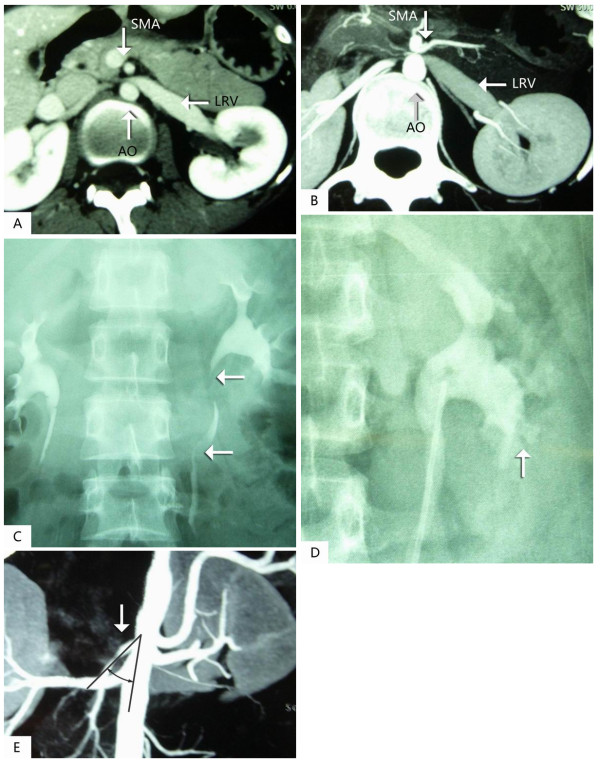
A (pre-contrast) and B (post-contrast), Computer tomography (CT) angiography demonstrates compression of the left renal vein between the aorta (A) and superior mesenteric artery (S) with the dilation of the distal part of the left renal vein on axial cuts. C, A retrograde pyelogram showed lobulated filling extravasate defects in calix at the lower pole of the left kidney. D, Excretory phase anterior volume rendered image shows tortuous, laterally displaced left proximal ureter (arrows) due to dilated varicose veins. E, A CT angiography demonstrates the acute angle between the aorta (A) and superior mesenteric artery (S) in this patient.
